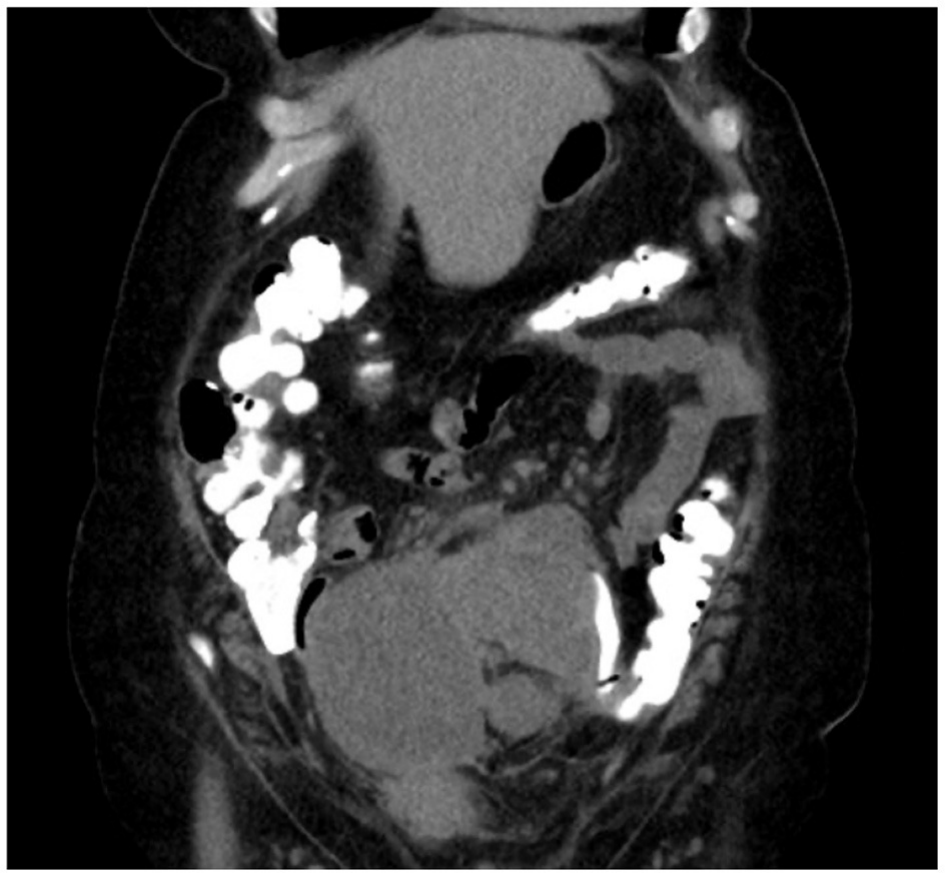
Coronal CT with mass measuring ~12.5 cm × 8.5 cm.
